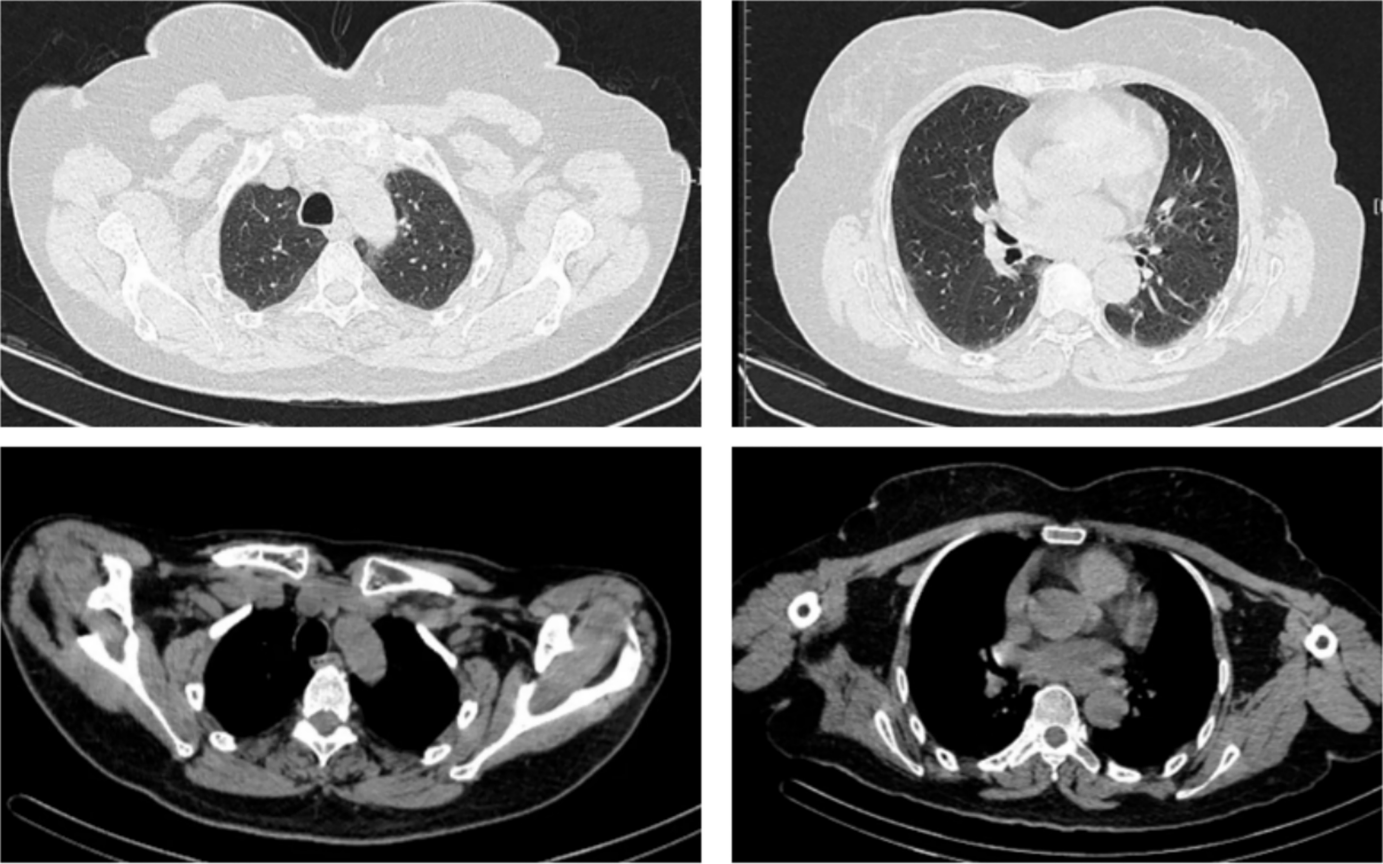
The non‐contrast chest CT scan on September 9, 2024, demonstrated complete resolution of the pulmonary mass following 4 cycles of bortezomib, dexamethasone, and thalidomide therapy.
